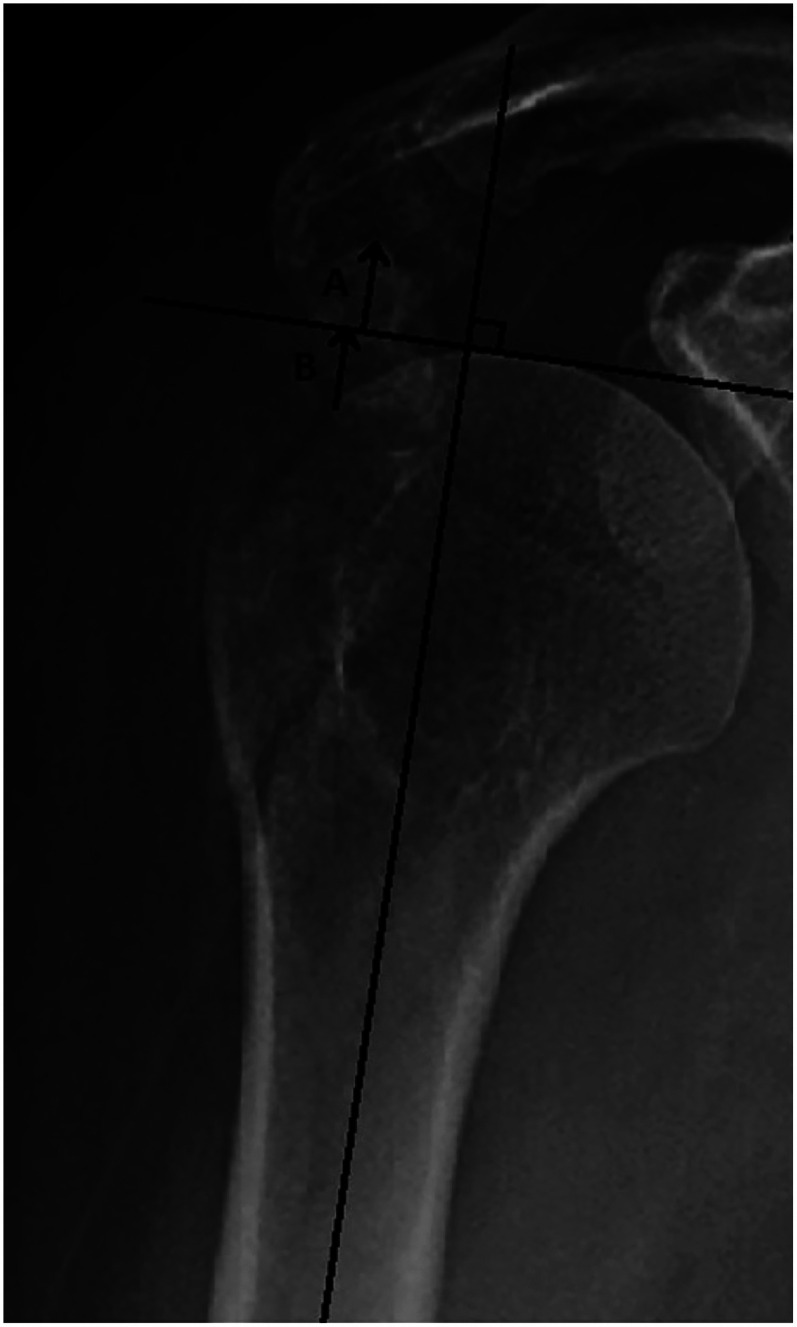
GT-ratio meassurements. The GT-ratio is used for superiorly displaced isolated GT fractures. GT-ratio = (A + B)/B. GT-ratio interpretation: < 0: Non-surgical. 0–0.5: Additional radiographs, i.e., CT. > 0.5 Surgical. Published with permission from the original author.^7^ GT: greater tuberosity; CT: computed tomography.
